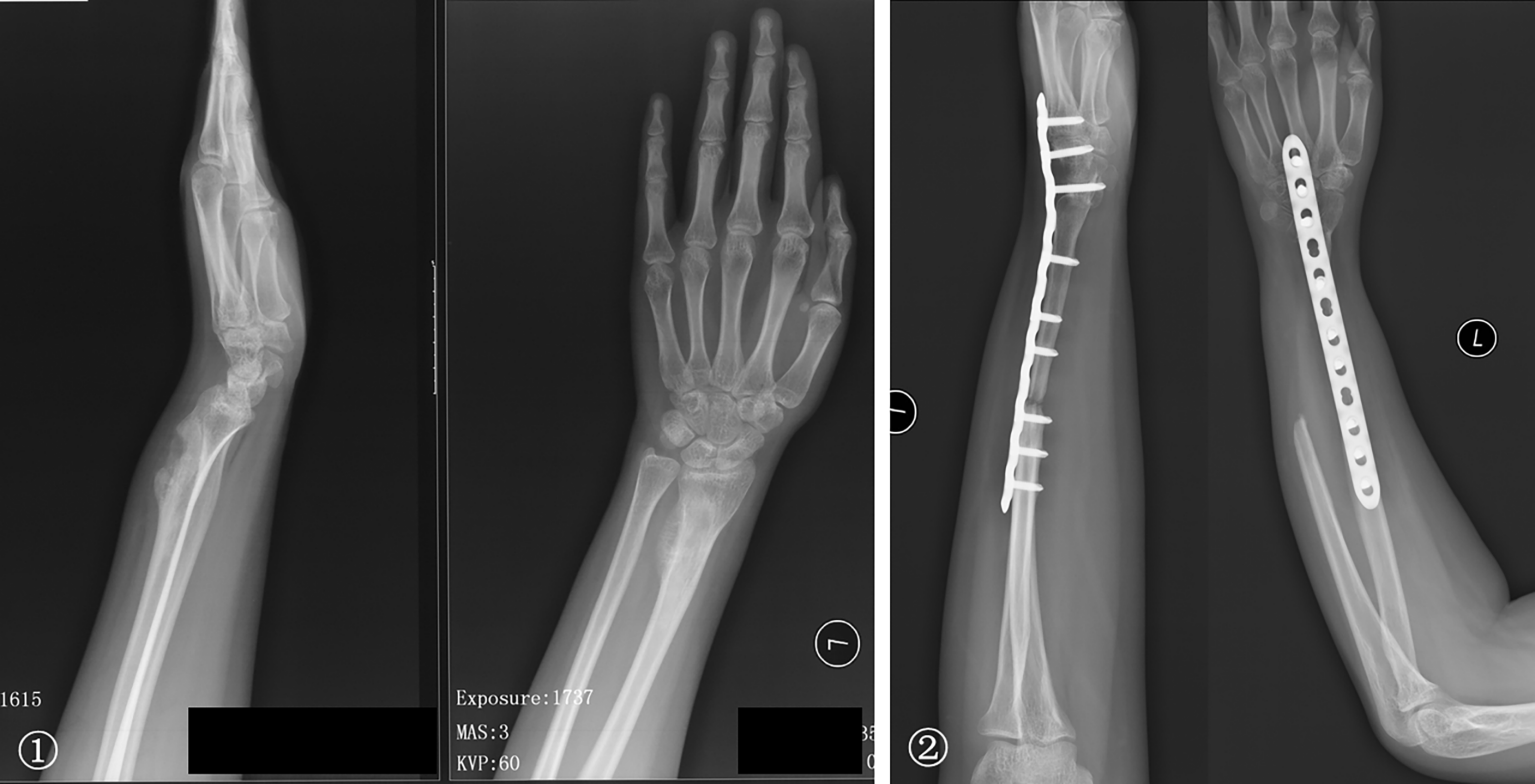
① The preoperative radiographs of a 19-year-old woman with low-grade central osteosarcoma of the distal radius. ② Treatments included a distal radius resection; ipsilateral ulnar osteotomy to replace the radial defect. Wrist joint fusion was performed; the satisfactory bone healing of the forearm and rotation function is shown 50 months postoperatively.
